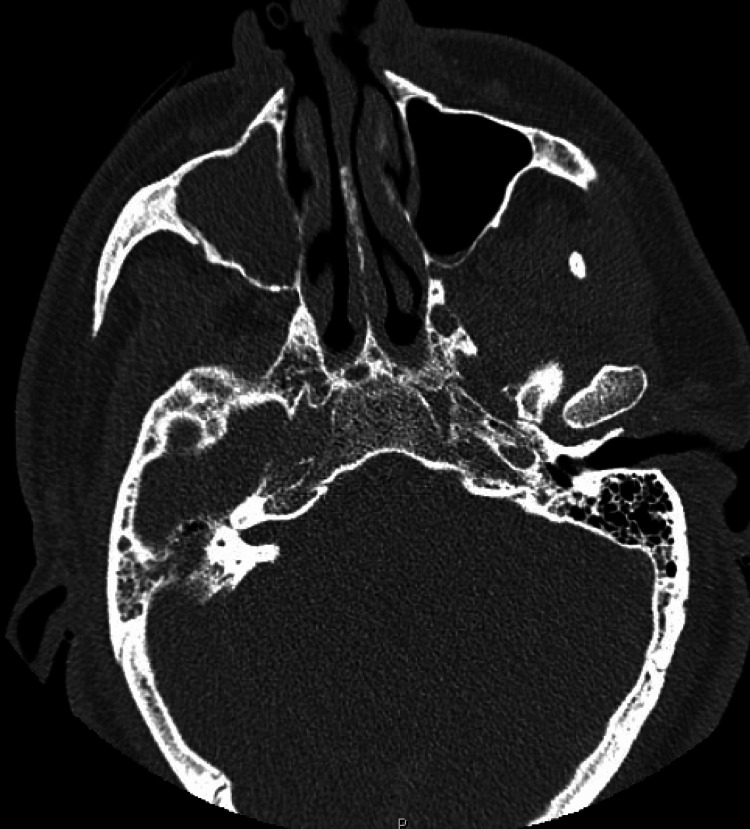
CT Sinus with contrast showing complete opacification of right maxillary air cells
